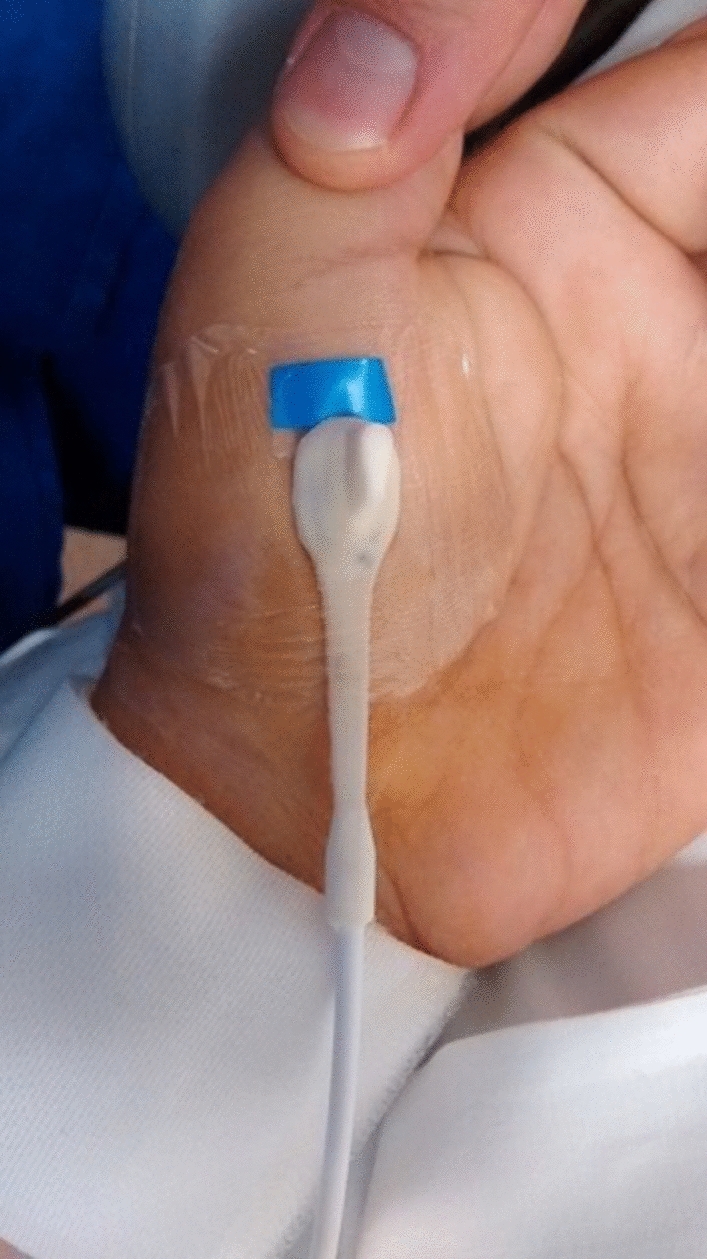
Laser-doppler flowmeter/white light spectrophotometry skin probe (LEA medical technology, Giessen, Germany) placing and fixation at thenar eminence.
